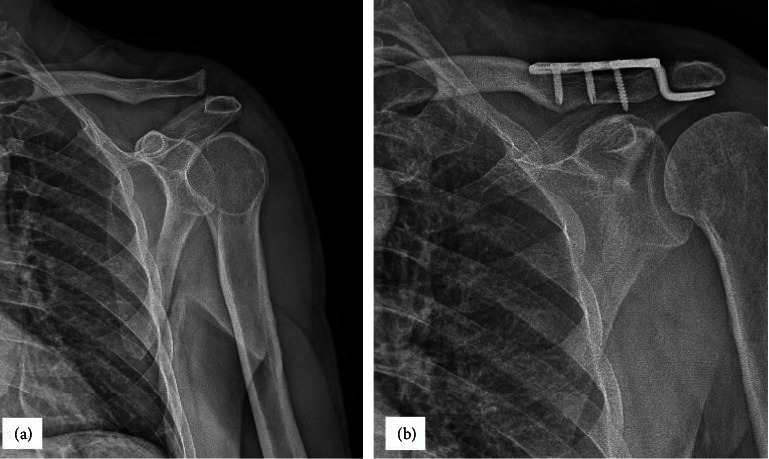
(a) The preoperative anterior and posterior view of a 32-year-old male patient with Rockwood type III acromioclavicular joint dislocation after a motorcycle accident injury. (b) 6-month postoperative plain radiograph showing good maintenance of joint reduction with hook plate fixation.
